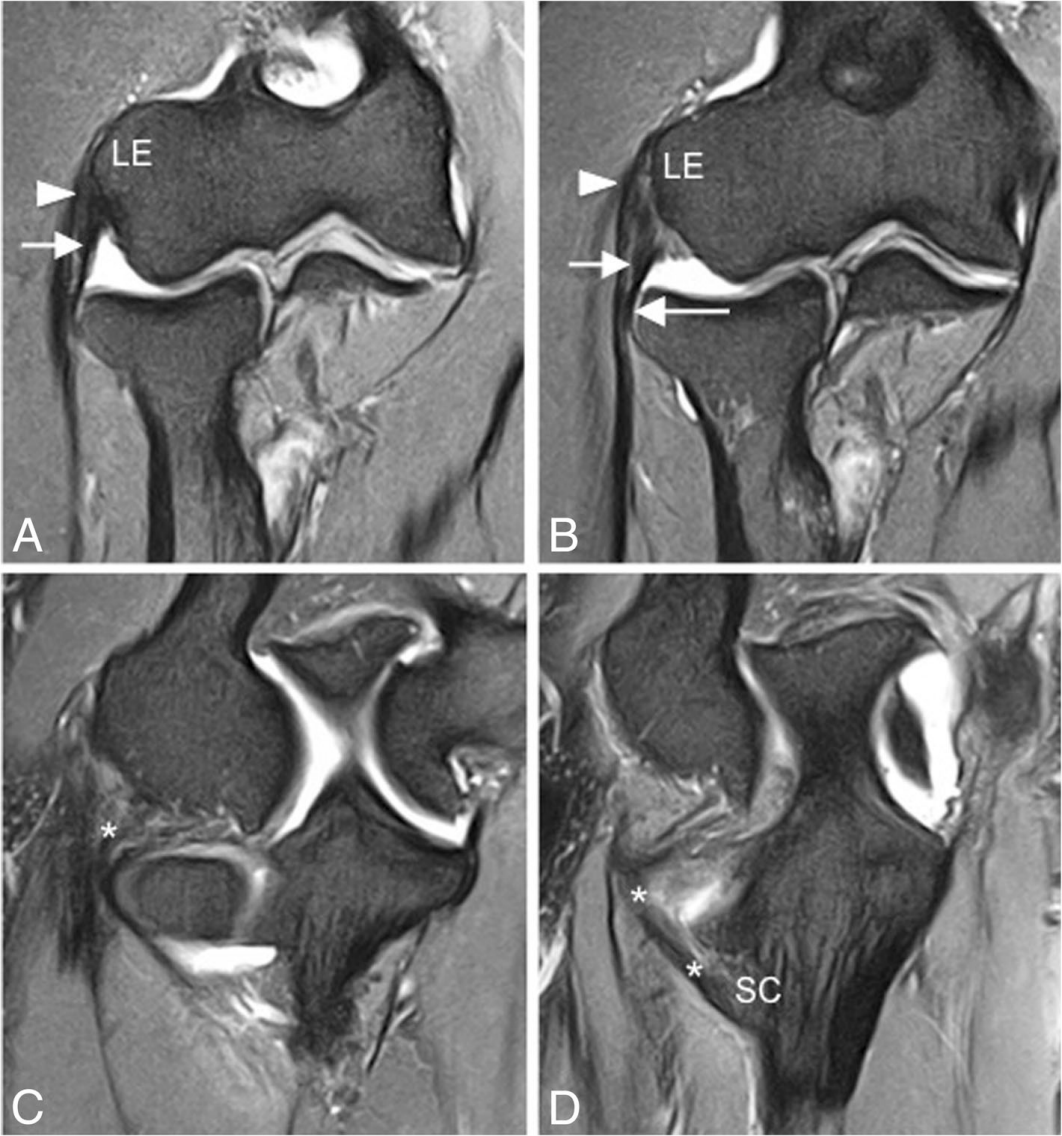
Consecutive coronal FS PD-weighted MRI (a–d) showing the intact lateral ulnar collateral ligament (white asterisks) and radial collateral ligament (short arrows). The lateral ulnar collateral ligament wraps around the posterior aspect of the radial neck. Annular ligament (long arrow). Common extensor tendon (white arrowheads). Lateral epicondyle (LE). Supinator crest of the ulna (SC)
